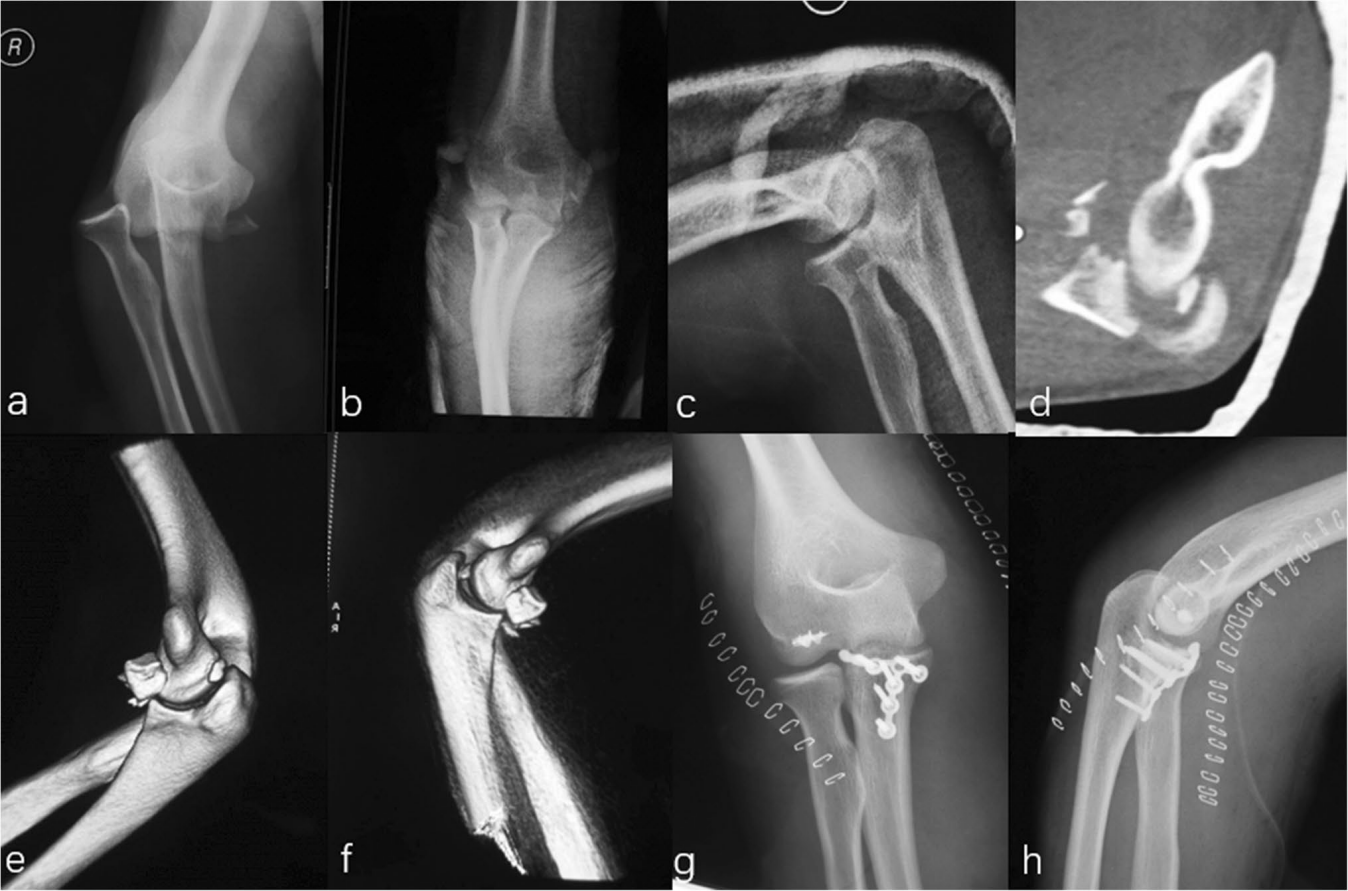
A 20-year-old male suffering a DDE and ulnar coronoid process fracture. Closed reduction was performed immediately (a–b). However, post-operative radiographs presented the ulnar coronoid process fracture was visibly large and lodged in the elbow joint (c–f). In addition, ORIF of the ulnar coronoid process and a repair of the LCL were also applied (g–h)
